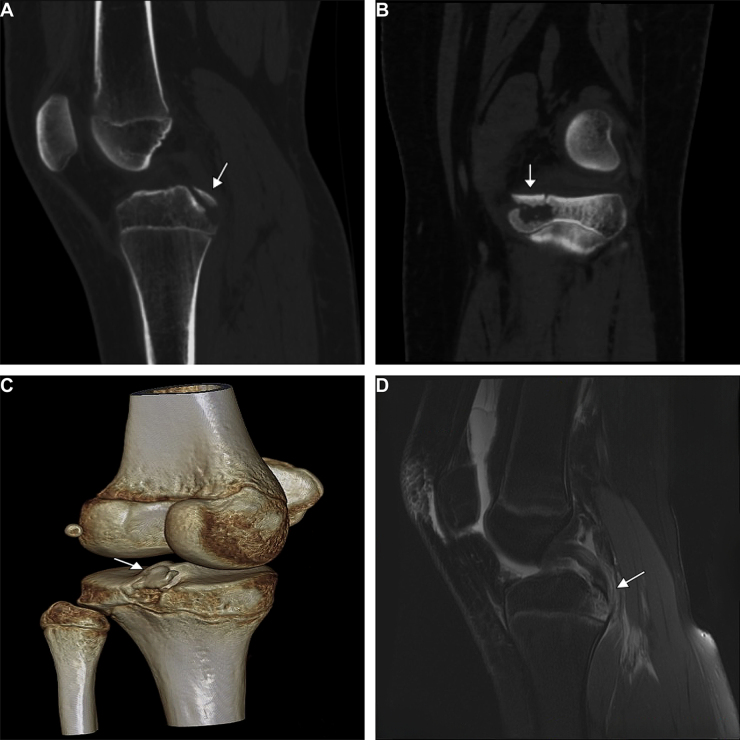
Preoperative imaging evaluation of the patient. (A) Sagittal computed tomography (CT) scan. (B) Coronal CT scan. (C) Three-dimensional CT scan. (D) Sagittal magnetic resonance imaging (MRI). Arrows highlight the avulsion fracture located at the tibial insertion of the posterior cruciate ligament (PCL).
